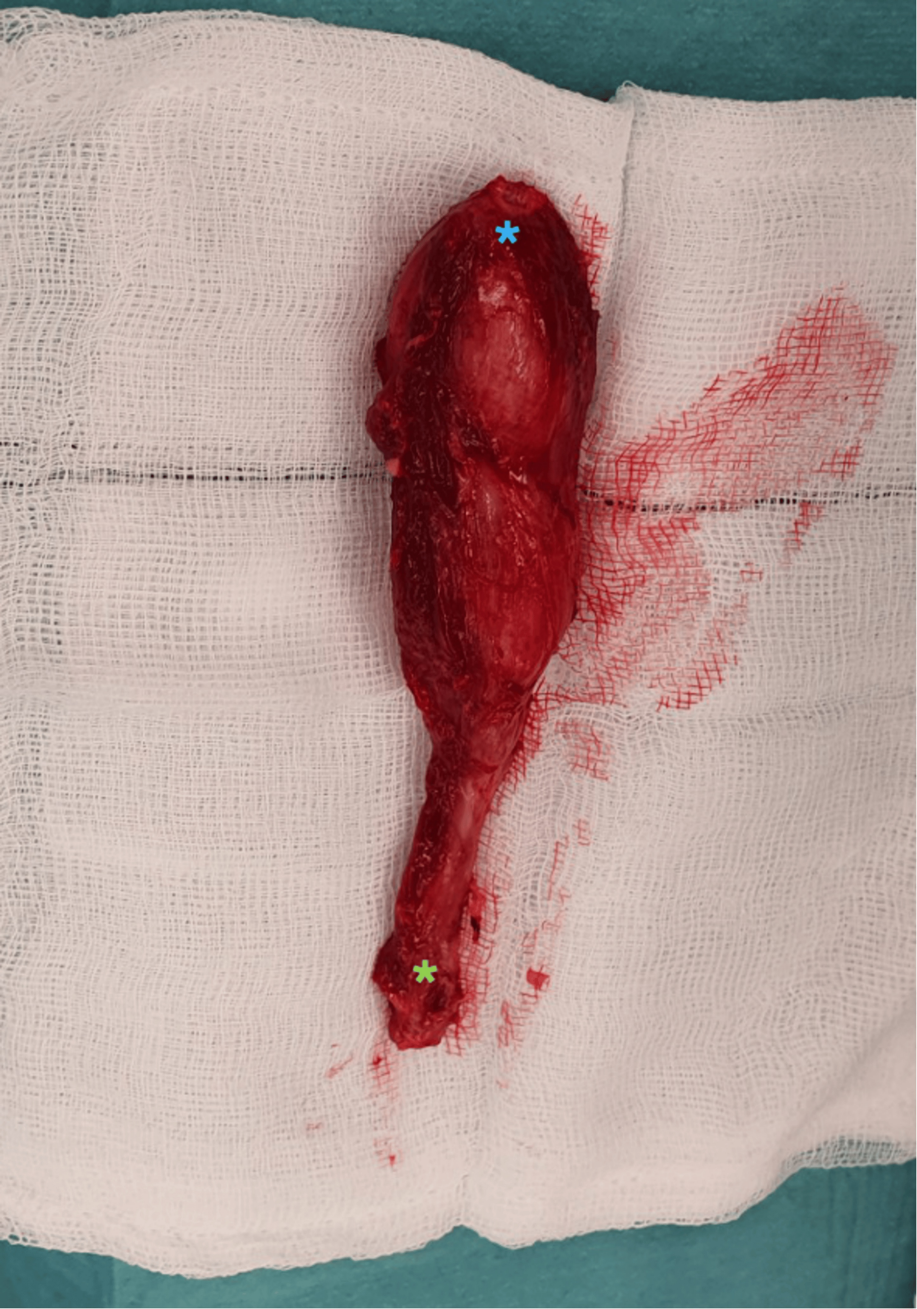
Excised pseudotumor. The pseudotumor was removed from the patient through the ilioinguinal approach. On MRI, it measured 7.5 cm × 3.3 cm × 10 cm. The blue asterisk indicates the proximal pole, while the green asterisk marks the distal pole of the pseudotumor.
